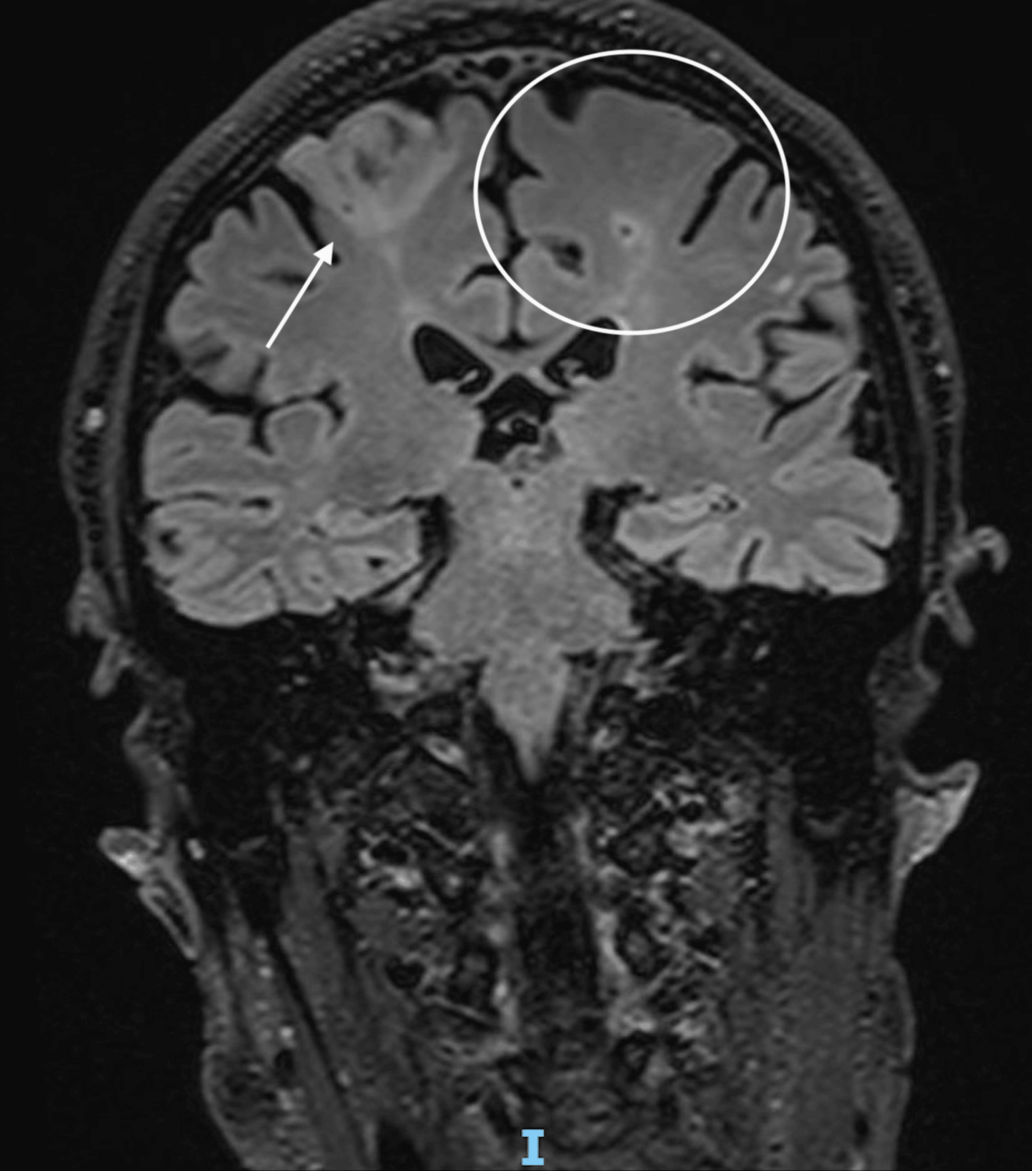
Brain CT showing bilateral evolving ischemic lesions
